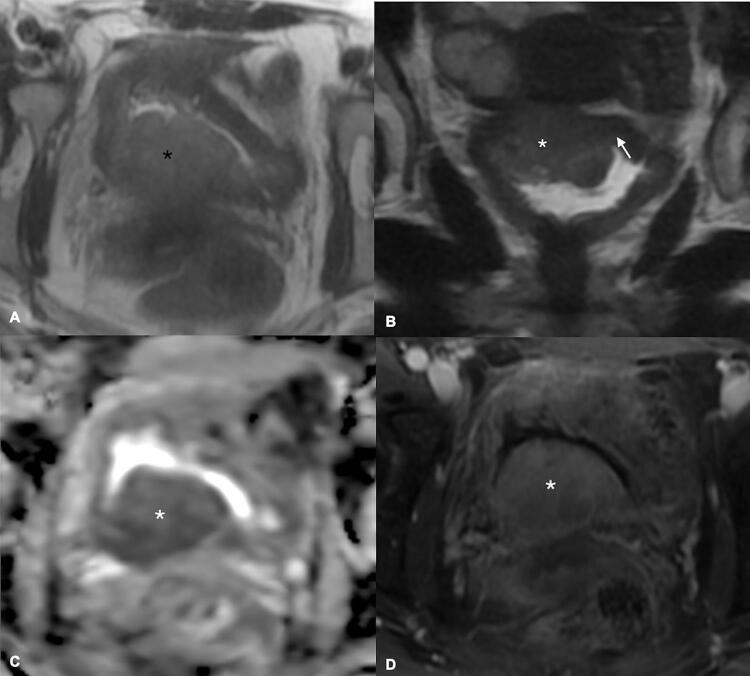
A 46-year-old, female, complains of frequency, urgency, and severe incontinence. A pelvic sonogram showed moderate to marked left hydronephrosis and asymmetric bladder wall thickening on the top portion of her bladder. A and B) Axial and Coronal T2-weighted MRI of the pelvis demonstrates a 4.4 x 3.6cm mass extending to muscle layer. C) ADC map in the axial plane, and D) T1 post-contrast, also in the axial plane, confirming that mass shows extension into the muscular layer. This was consistent with VI-RADS 4, confirmed after surgery.
